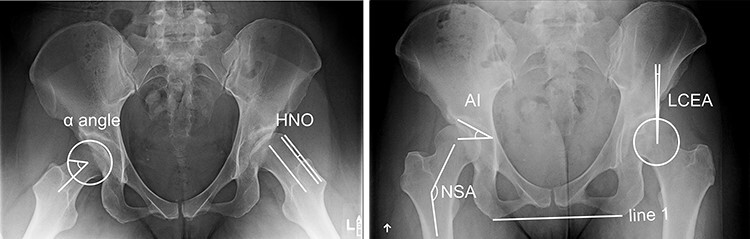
Radiographic descriptors of the femoral head and acetabulum. The α angle was measured as the angle between the femoral neck axis and the point of beginning asphericity. HNO was the distance between a line parallel to the femoral neck axis and tangent to the anterolateral neck, and a second parallel line tangent to the anterolateral head. NSA on the anteroposterior view was the angle between the femoral neck axis and the long axis of the femur. AI was the angle between a line parallel to the inter-ischial-tuberosity line (line 1) at the inferior aspect of the acetabular sourcil and a second line from the inferior to lateral aspects of the sourcil. LCEA was the angle between a line perpendicular to line 1 originating from the center of a femoral head best-fit circle and a second line from the center of the circle to the lateral aspect of acetabular sourcil.
